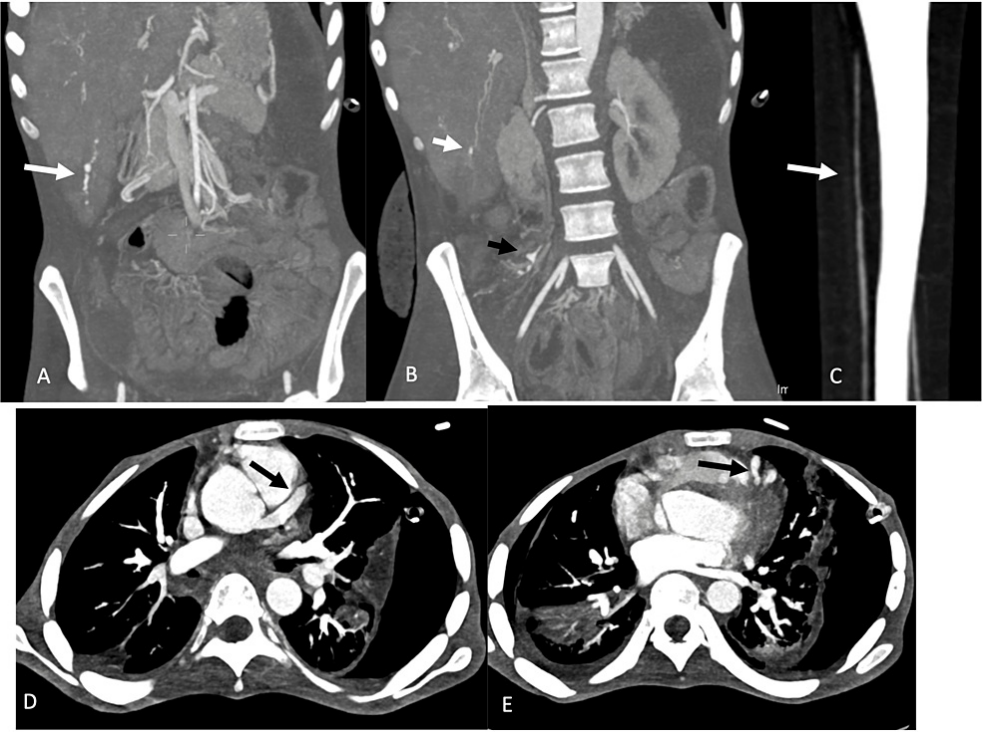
CT angiogram of the chest, abdomen, and lower extremity runoffs follow-up after two months; performed as the patient complained of toe pain. (A, B) Coronal maximum intensity projection (MIP) images of the abdomen showing abnormal beaded appearance of the right hepatic artery with micro-aneurysms (white arrows) and similar appearance to the ileocolic artery (black arrow). (C) Coronal MIP image of the right leg showing subtle arterial luminal irregularity of the right anterior tibial artery. This is less apparent than the visceral arteries in A and B. (D, E) Axial MIP images of the chest showing saccular dilatation of the proximal left anterior descending artery (D) and distal areas of beaded appearance (E).
